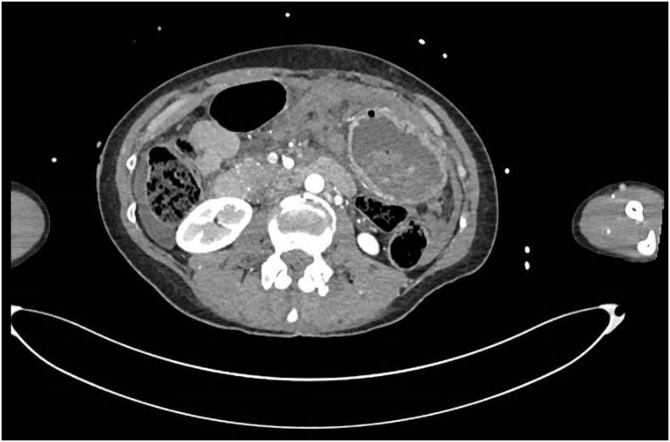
Axial CT demonstrating free gastric content in the peritoneal cavity.
